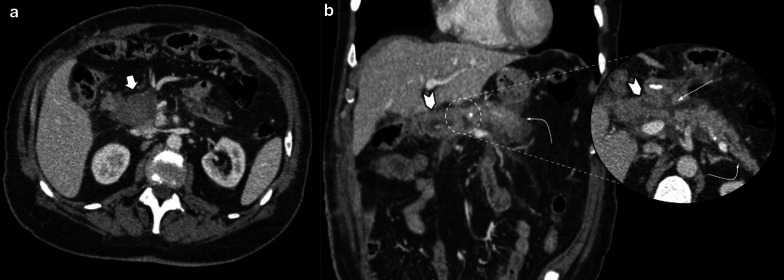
Pancreatic fistula, (a, b) Axial and coronal portal venous phase CT scan, respectively. 72-year-old male patient with pancreaticoduodenectomy due to neuroendocrine tumor, during second-month control CT scan. Homogeneous collection (big arrow) in surgical bed. That collection impresses to be in contiguity with the PJ (straight arrow). Normal pancreatic stump (curved arrow) and afferent loop (arrowhead) are also noted
